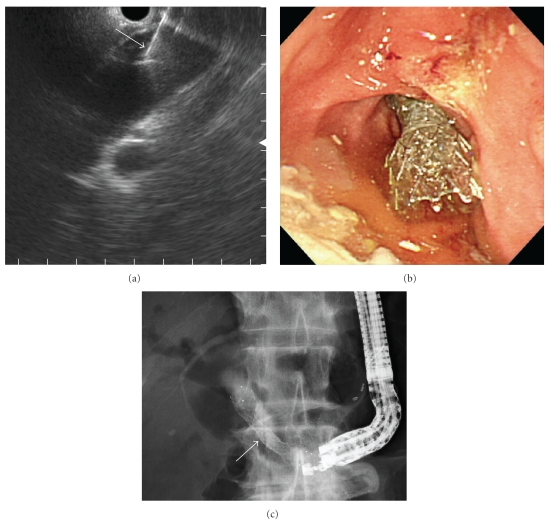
Endosonography-guided biliary drainage ((a) endosonography; (b) duodenoscopy; (c) fluoroscopy). Using a convex linear-array echoendoscope, puncture of the extrahepatic bile duct via the duodenal bulb was performed with a 19G needle (arrow) (a). A fully-covered metal stent (c, arrows) (covered ZEOSTENT), 6 cm in length and 1 cm in diameter, was placed via the puncture tract (b, c).
